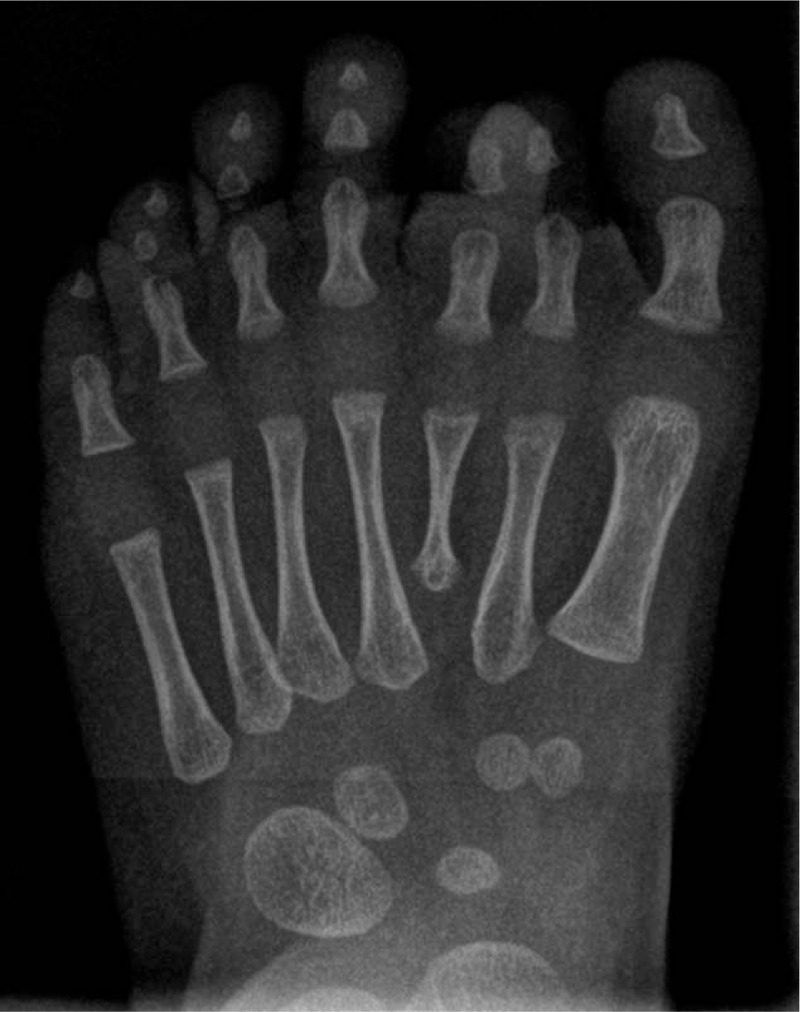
Foot radiographs revealed the presence of 7 complete rays (frontal plane).
